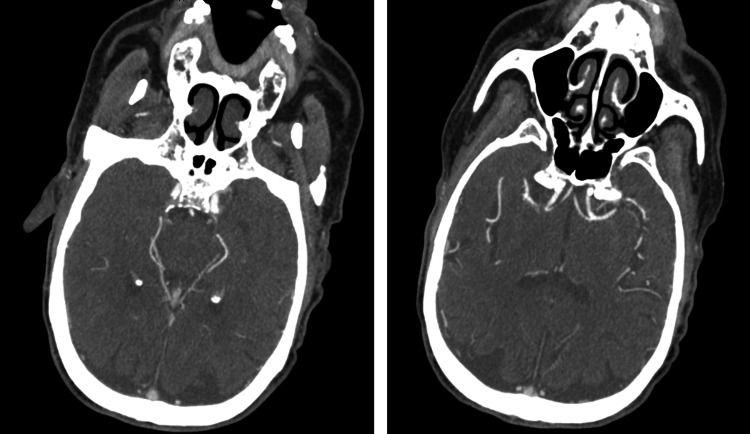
CT angiogram showing a patent Circle of Willis, anterior cerebral artery, and middle cerebral artery. CT, computed tomography
